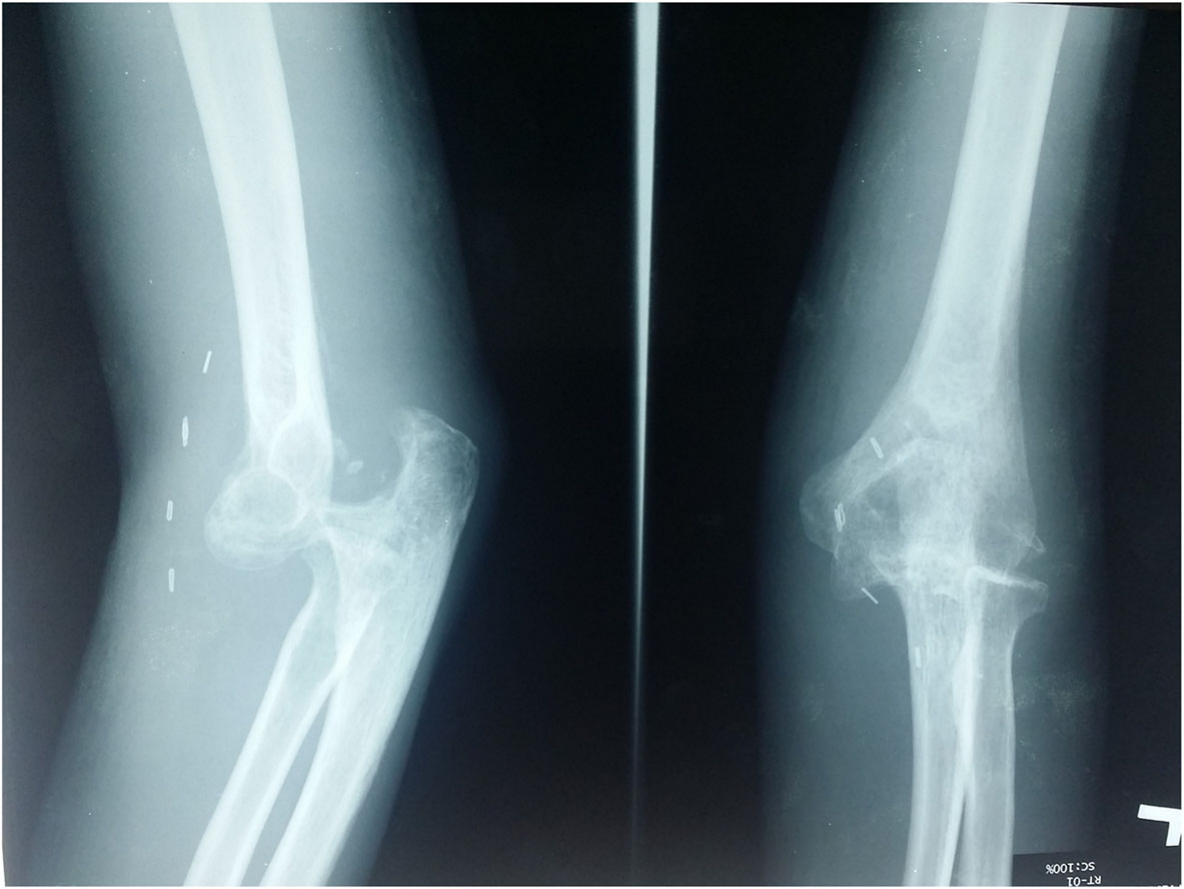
Radiograph of left elbow before closed manipulative reduction
